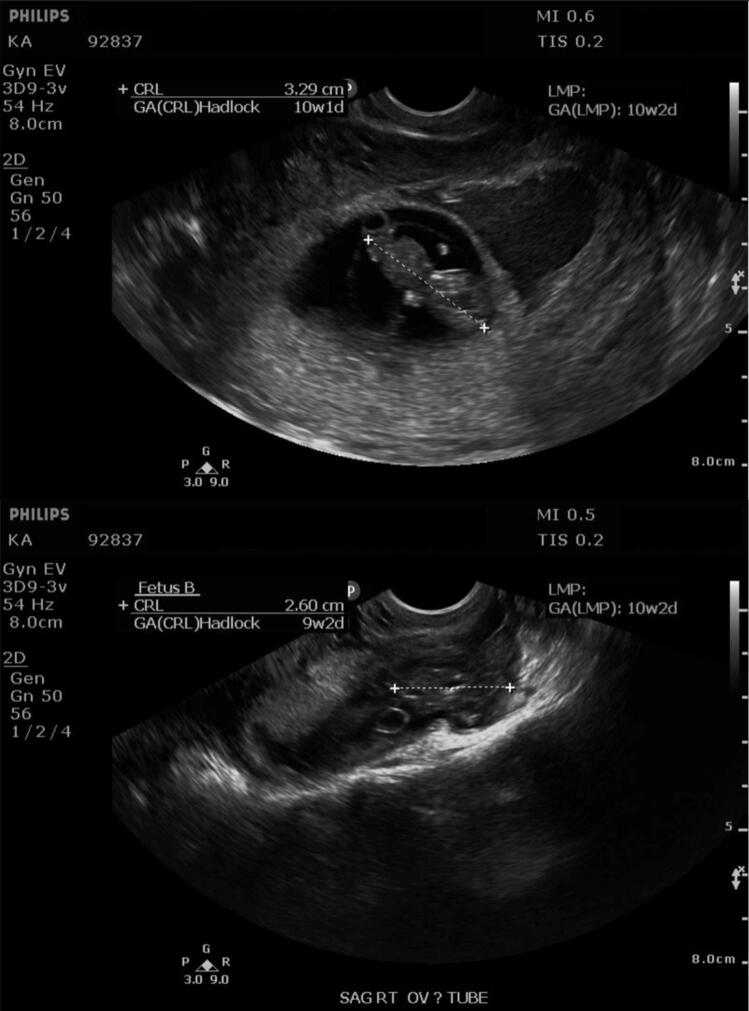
Transvaginal ultrasound at 10 weeks 2 days of gestation after recurrence of painless bleeding. Top: Single intrauterine pregnancy with CRL of 3.29 cm and adjacent yolk sac. Bottom: Right adnexa displays a second gestational sac, “Fetus B", with CRL of 2.60 cm corresponding to 9 weeks 2 days of gestation by Hadlock dating, consistent with an ectopic pregnancy.
